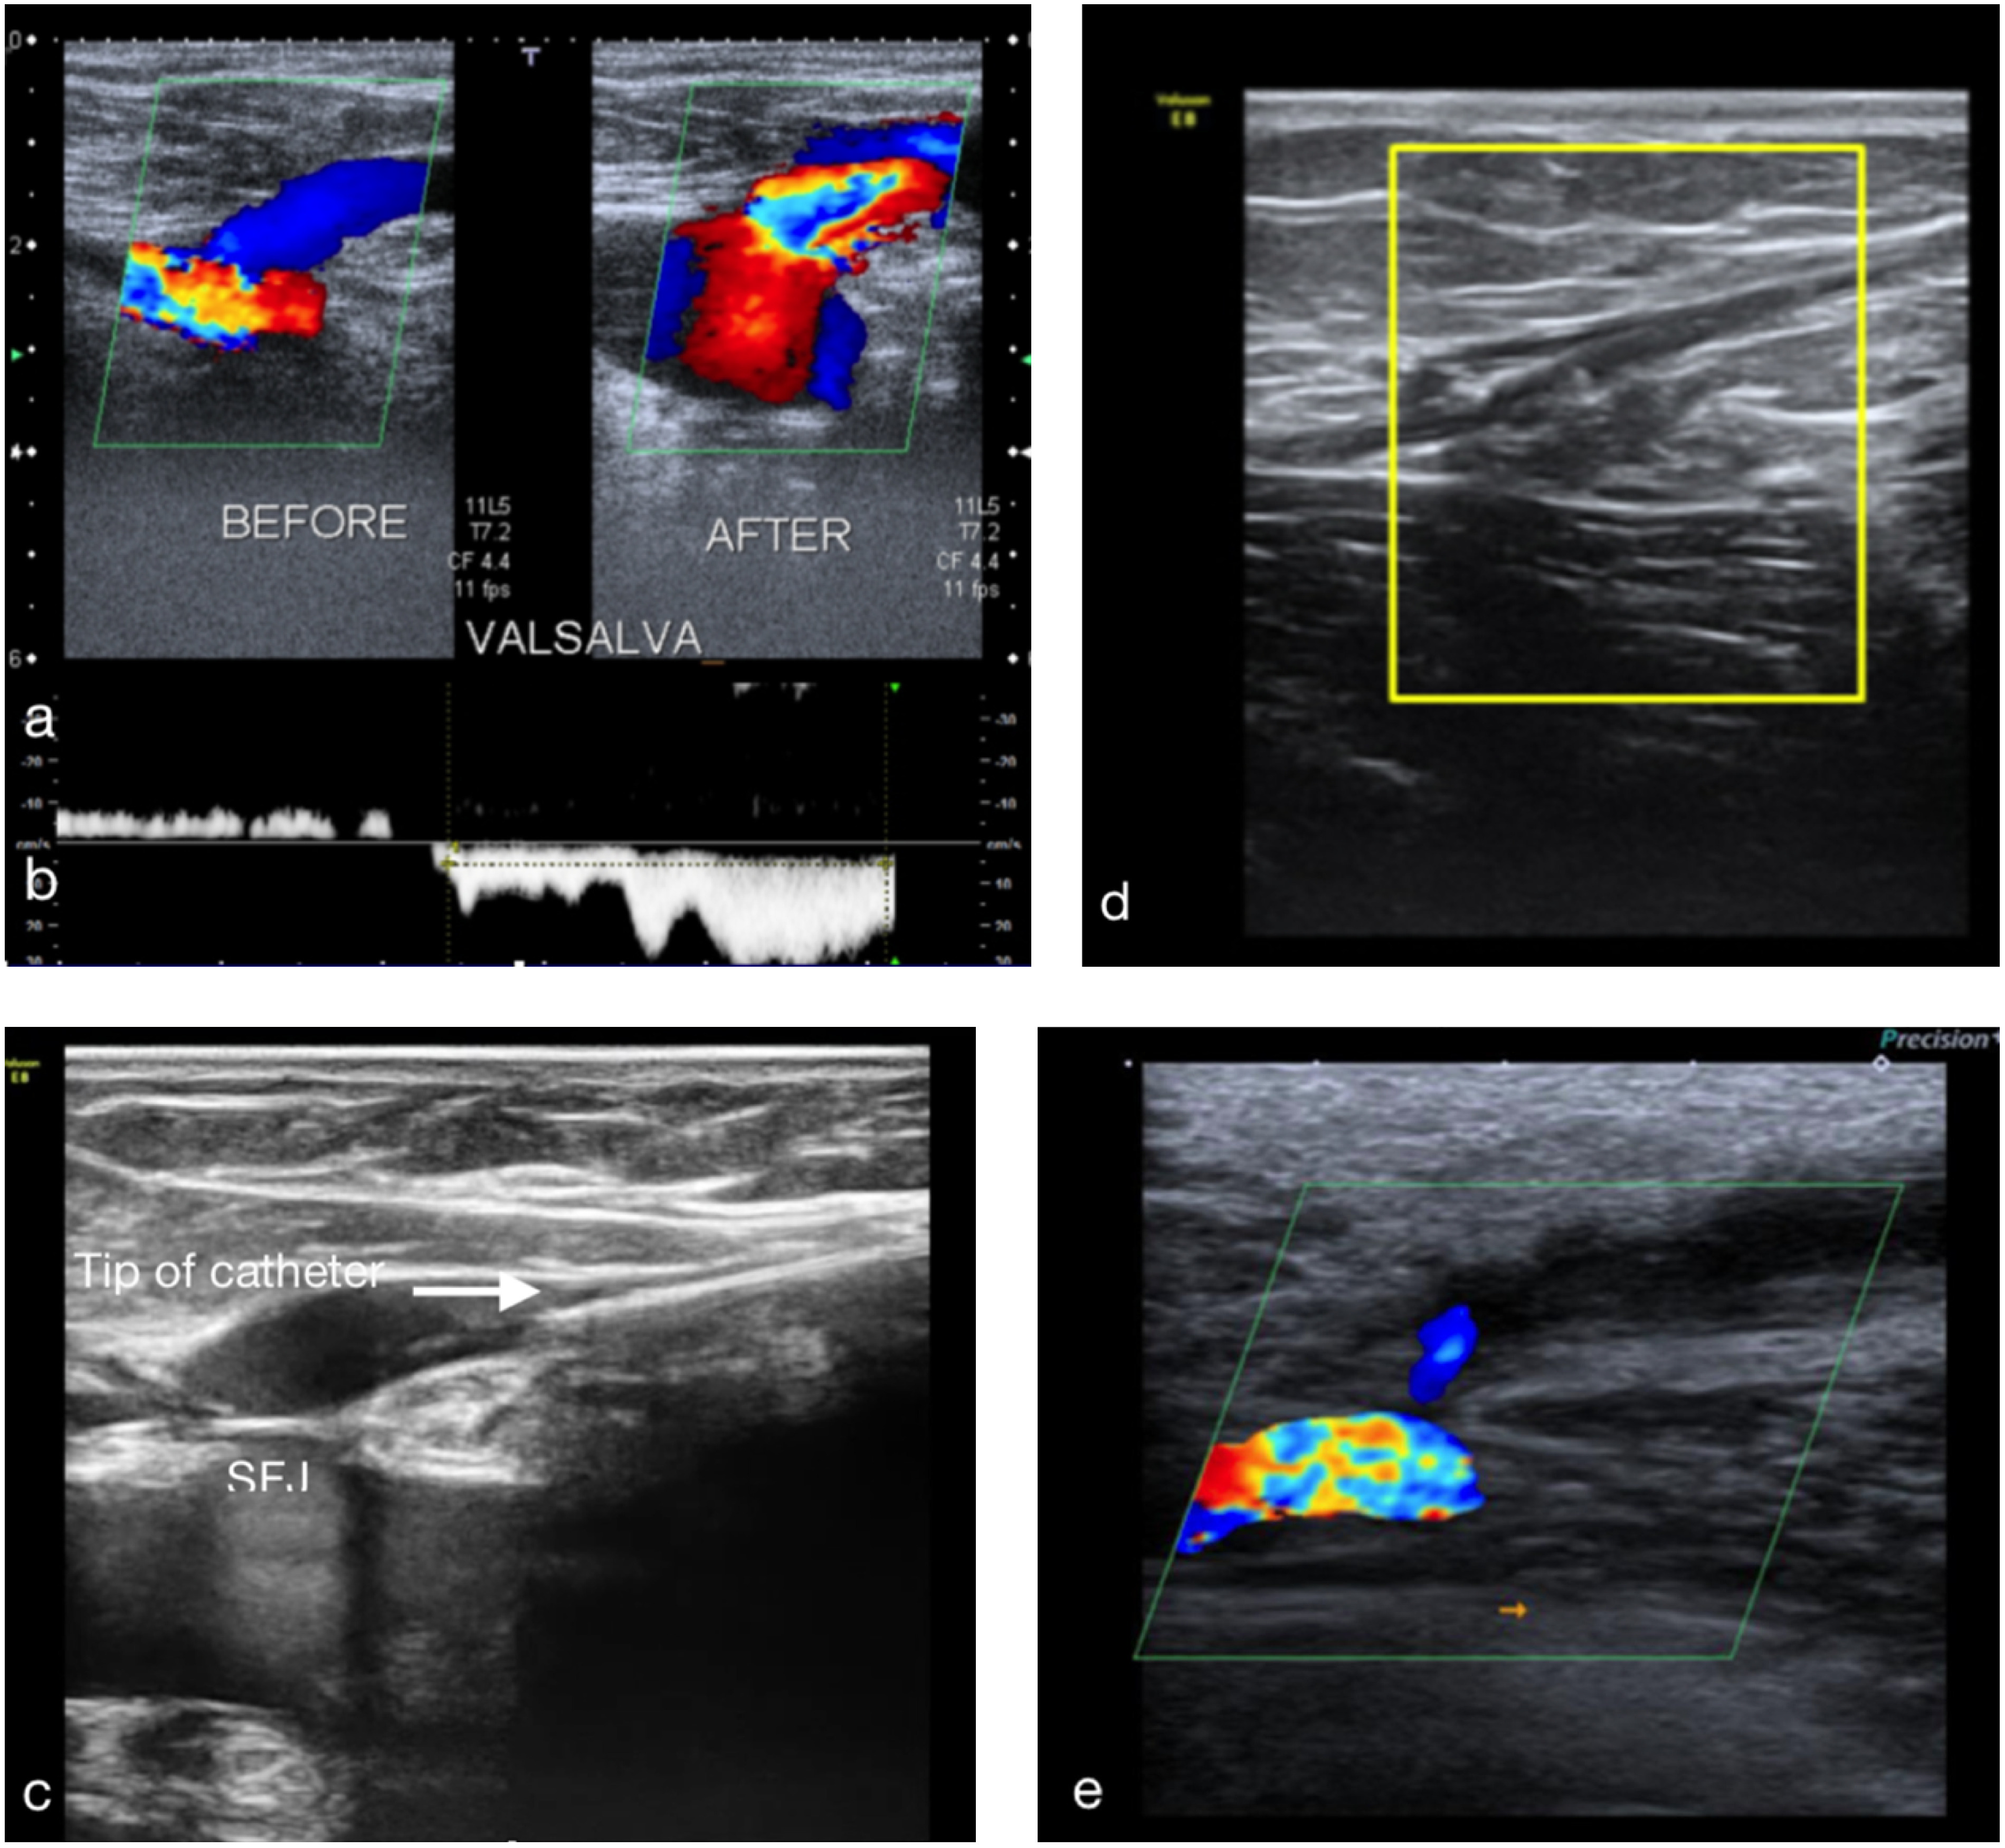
Fig. 1 Radiofrequency ablation (RFA). (a)–(c) Case 1-A 46-year-old male with refluxing right great saphenous vein (GSV). Pathologic reflux after the augmentation maneuver with Doppler image showing change in color, increase in caliber of GSV (a); reflux time >0.5 s observed on Doppler (b); tip of RFA catheter seen approaching the saphenofemoral junction (SFJ) (>2 cm away from SFJ) (c); (d)–(e) Case 1 continued. Immediate post-RFA ultrasound shows thrombosed GSV (d); Repeat Doppler at 24 h confirms the absence of blood flow in the GSV (e).
